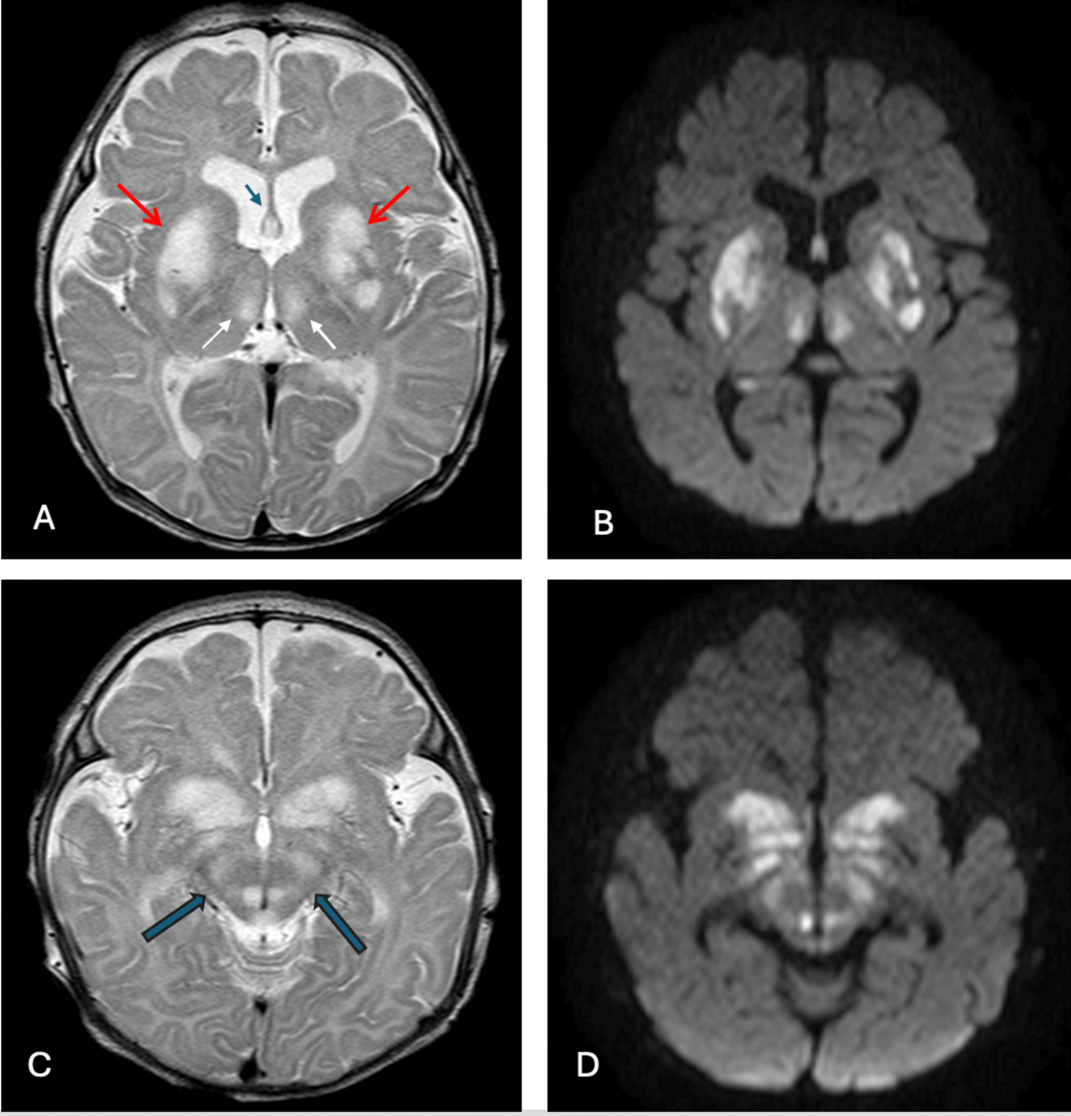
Brain magnetic resonance imaging findings. (A) Axial T2-weighted sequence demonstrates bilateral symmetric hyperintense signal abnormalities within the lentiform nuclei (red arrows) and medial thalami (white arrows). Additional areas of T2 hyperintensity are noted in the perirolandic cortex, fornix (thin blue arrow), hypothalamus, bilateral hippocampi, and splenium of the corpus callosum (regions not shown). (B) Corresponding axial diffusion-weighted imaging shows restricted diffusion in the same anatomical distributions. (C) Axial T2-weighted image at the midbrain level reveals symmetric hyperintense signal changes involving the cerebral peduncles, substantia nigra, and periaqueductal gray matter (thick blue arrows). (D) Axial diffusion-weighted imaging at the midbrain level confirms restricted diffusion within these brainstem structures, consistent with acute metabolic injury.
